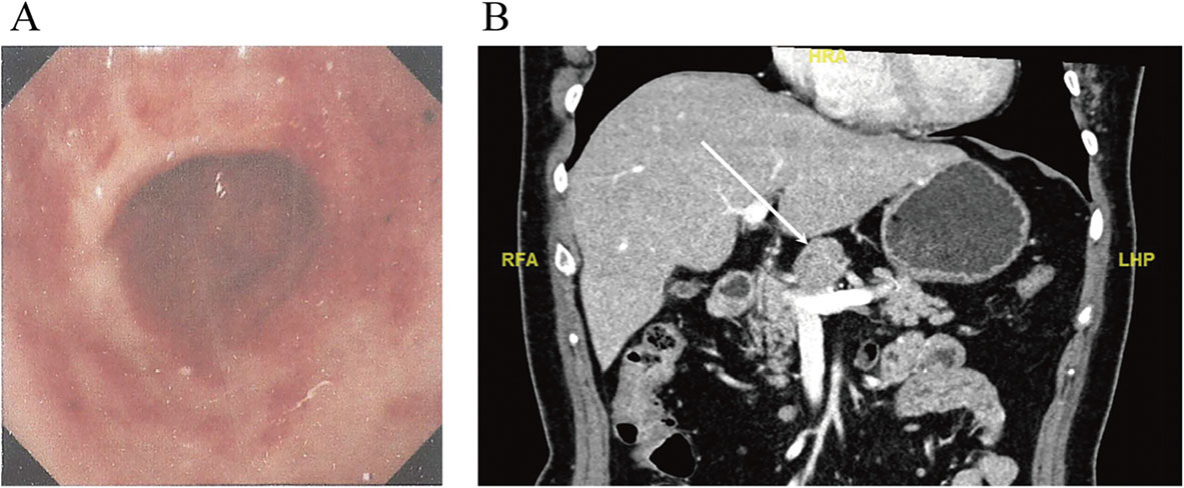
The first preoperative tests. a The first gastroscopy that was taken at another hospital (scanned version of printed report). Slight superficial mucosal erosion of the gastric antrum. b The contrast-enhanced abdominal CT. A solitary nodule above the neck of the pancreas, size 29 mm × 27 mm, labeled by the white arrow
